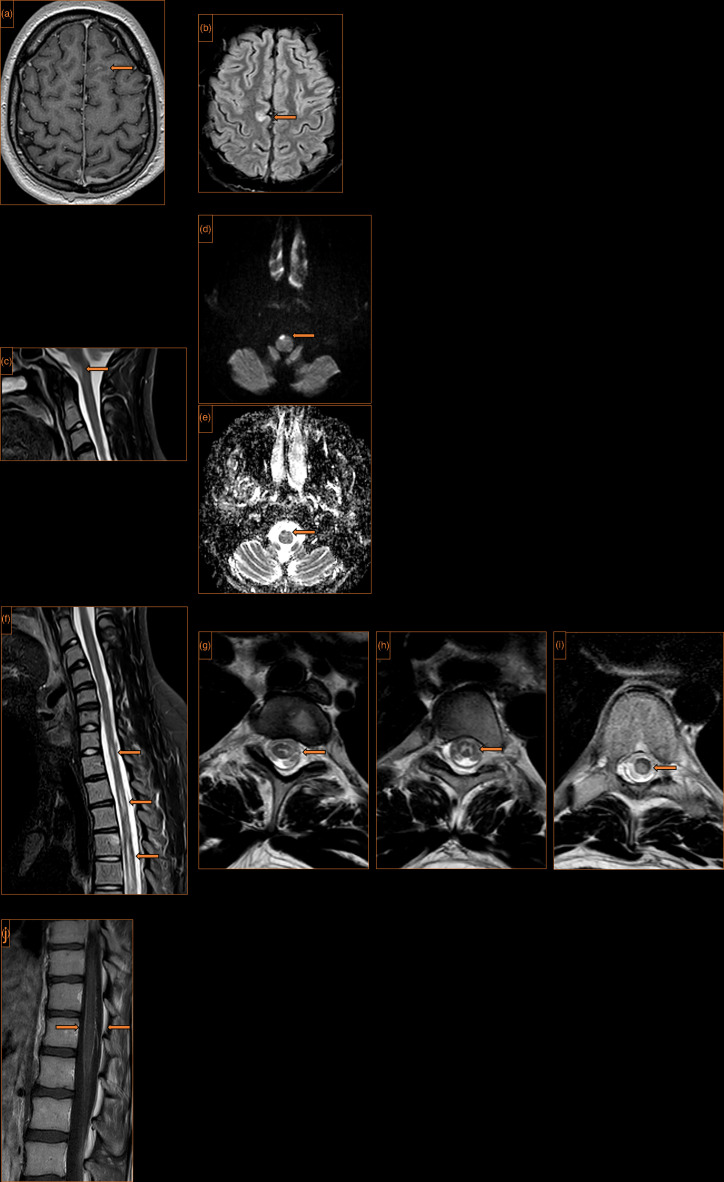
MRI of the head and spine. (a) Axial T1 post-gadolinium contrast of the brain. Focal leptomeningeal enhancement high left frontal lobe (arrow). (b) Axial T2 fluid-attenuated inversion recovery of the brain. A focal high signal lesion at the parafalcine cortex right frontal lobe (arrow). (c) Sagittal T2-weighted images of the craniocervical junction. A high signal lesion in the anterior medulla oblongata (arrow). (d) Axial diffusion-weighted imaging of the craniocervical junction. Restricted diffusion within the lesion (arrow). (e) Complementary low signal on the apparent diffusion coefficient map (arrow). (f) Sagittal T2-weighted images of the cervicothoracic spine. Multiple focal high signal lesions within the spinal cord, measuring up to two vertebral levels in length (arrows). These lesions are associated with cord swelling and did not enhance after contrast. (g−i) Axial T2-weighted images at three levels of the dorsal spine. Heterogeneity of location with the cord substance, with some predilection for grey matter (arrows). (j) Sagittal T1 of the lumbar spine with intravenous gadolinium contrast. Florid leptomeningeal enhancement over the surface of the conus (arrows).
